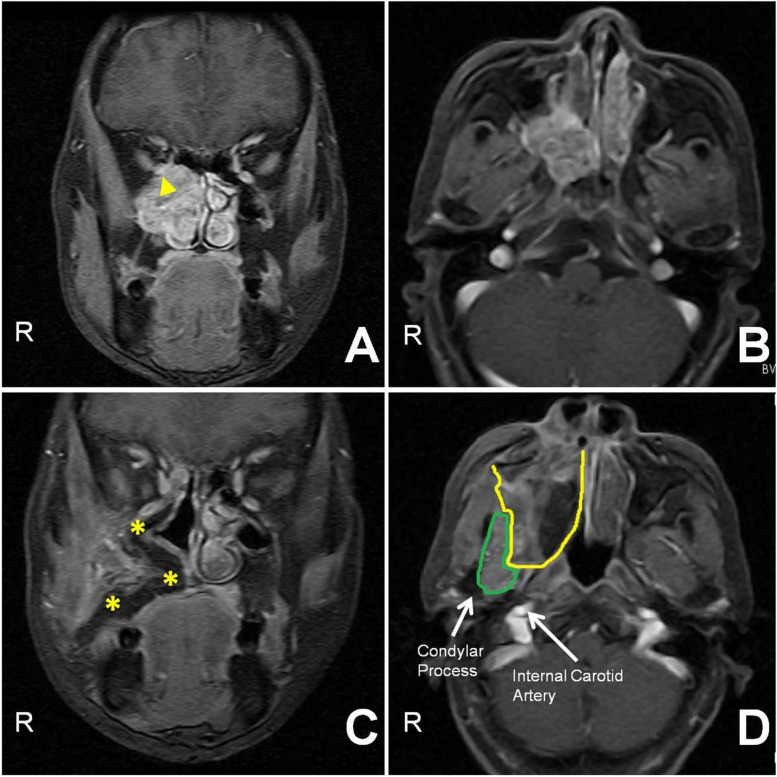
(A,B) Preoperative contrast-enhanced coronal and axial T1-weighted MR images show that a tumor (triangle) occupied the PPF, invading the orbit and nasocavity. (C) Thirteen-month postoperative contrast-enhanced coronal T1-weighted MR image demonstrates that the flap (asterisk) supported the orbital contents and covered the defects without recurrence. (D) The postoperative contrast-enhanced axial T1-weighted MR image shows that the maxillary-swing approach failed to resect the lateral part of the infratemporal fossa (referred to as the “blind spot,” bordered in green; the flap is encircled by the yellow line).
